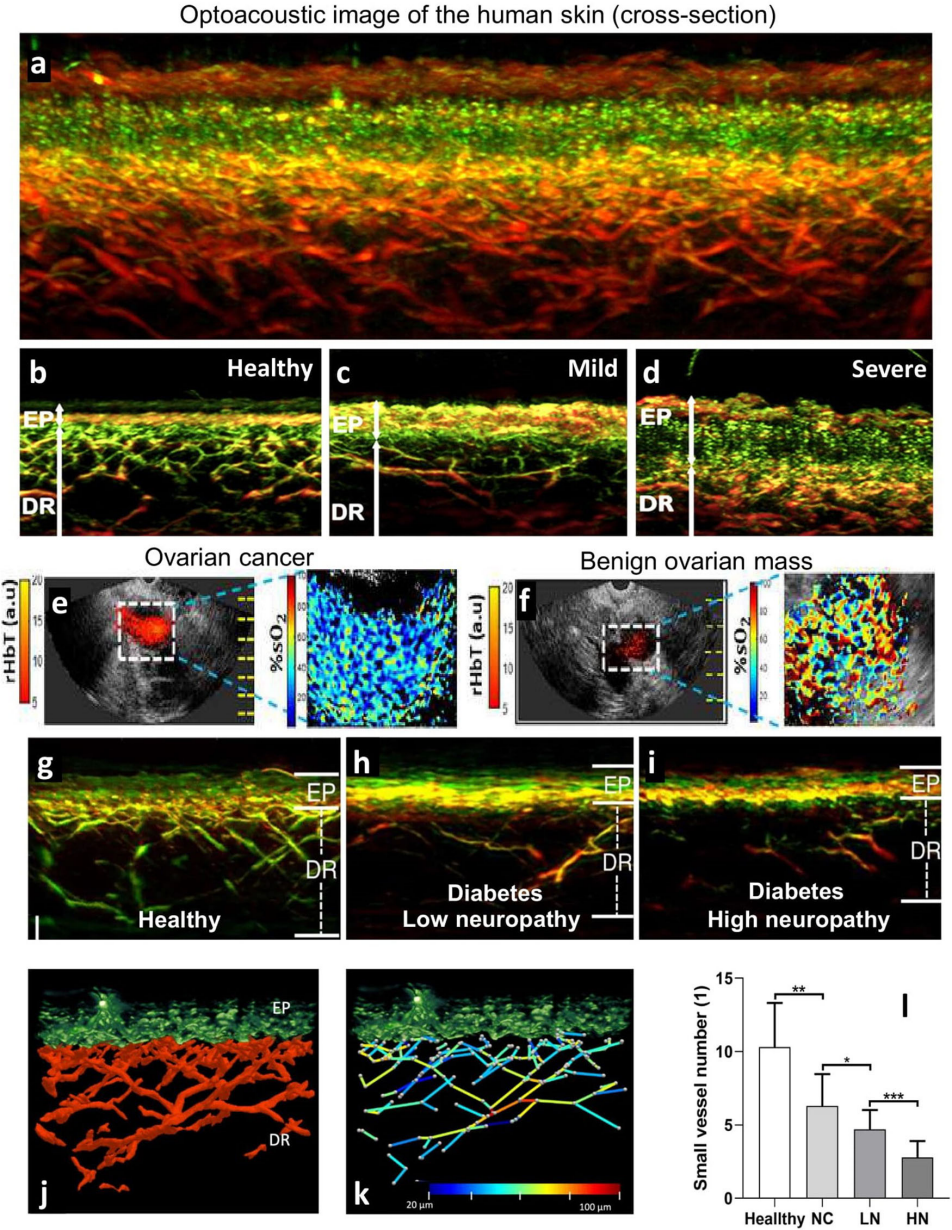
Representative in vivo mesoscopic images of healthy volunteers or patients with different diseases. a Cross-sectional Raster-Scan Optoacoustic Mesoscopy (RSOM) image of the human skin from a healthy volunteer, demonstrating the detail resolved at 532 nm. Green or red colors represent the high or low spatial frequencies, i.e., the finer or coarser vasculature, respectively. Fine capillary loops are seen at the epidermis as “dot”-like structures with resolutions that may reach 7 micrometers. Adapted from Aguirre et al.^20^ with permission. b–d Representative cross-sectional RSOM images of varying eczema severities. EP is the epidermal layer, whereas DR is the dermal layer. b Healthy volunteer, c patient with mild eczema, and d patient with severe eczema. Reprinted from Yew et al.^59^ with permission from Elsevier. e–f Co-registered ultrasound (US) and oxygenation saturation (%sO_2_) in ovaries with a e malignant or a f benign mass. Adapted from Amidi et al.^73^ with permission from John Wiley and Sons. g–i Example of OptA skin mesoscopy detecting systemic disease with RSOM skin images from a healthy volunteer and two diabetic patients with low and high score neuropathy. Adapted from He et al. under a CC-BY 4.0 license^49^. Segmentation of such images (j) could lead to an extraction of multiple features (k) that could classify diabetes progression. EP epidermis, DR dermis. Adapted from He et al. under a CC-BY 4.0 license^61^. l NC: Diabetes patients with no complications, LN: diabetes patients with low-grade neuropathy, HN: diabetes patients with high-grade neuropathy. Adapted from He et al. under a CC-BY 4.0 license^49^.
